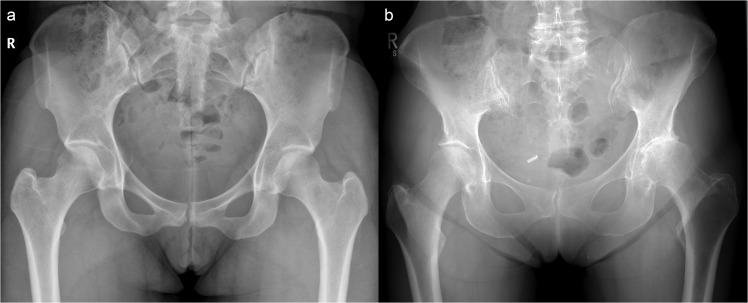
Anteroposterior radiographs of the pelvis in two adult females. The individual in (a) has no evidence of radiological osteoarthritis on either side (KL grade 0); however, the individual in (b) has marked disease asymmetry, with no radiological disease in the right hip (KL grade 0), but moderate disease in the left (KL grade 3).
